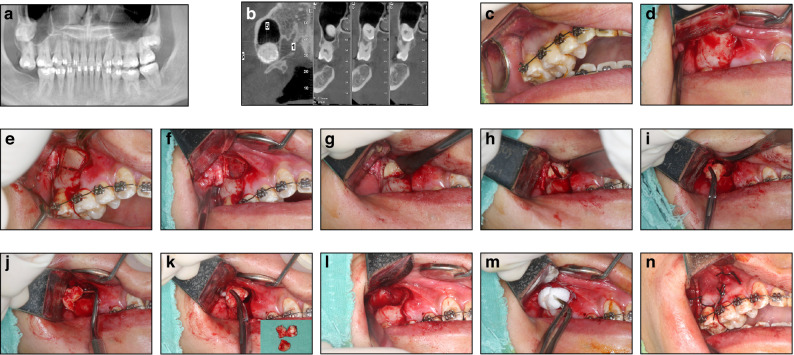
Extraction of an impacted maxillary third molar adjacent to the maxillary sinus via the submaxillary sinus membrane space approach. a, b CBCT images indicating the position of the impacted third molar. c The molar is invisible in the oral cavity. d Design of the incision and elevation of the mucoperiosteal flap. e The bony window created via piezosurgery. f Elevation and reflection of the buccal bone flap to reveal the underlying maxillary sinus membrane. g Elevation of the maxillary sinus membrane resulting in tooth exposure. h–k Removal of the impacted tooth in pieces following tooth sectioning through the bony window. l Intact maxillary sinus membrane. m Copious irrigation and placement of a collagen plug sponge into the sub-MSM space for hemostasis with the aim of preventing oroantral communication. n Primary suturing of the wound
